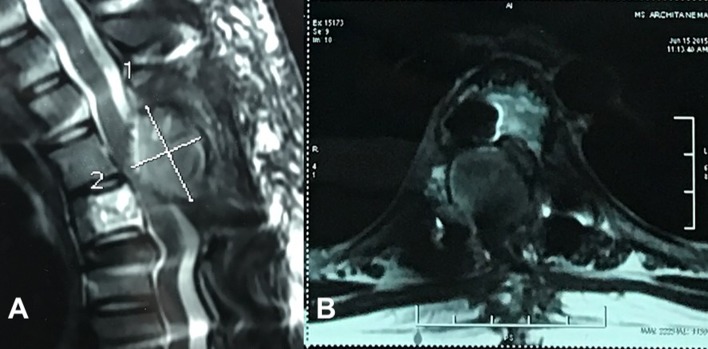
MRI after second recurrence showing Epidural compressive mass (cross-hairs) from the right side with underlying Myelomalacia of the spinal cord with ventral displacement on sagittal (A) and axial scans (B).
